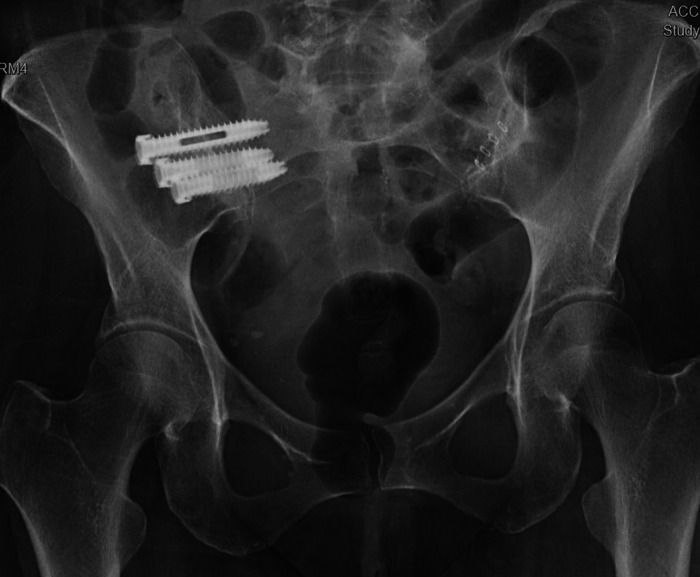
Post-implantation X-rays demonstrating appropriate placement of the hydroxyapatite-coated titanium implants through the right SI joint (from (36), used with permission).
